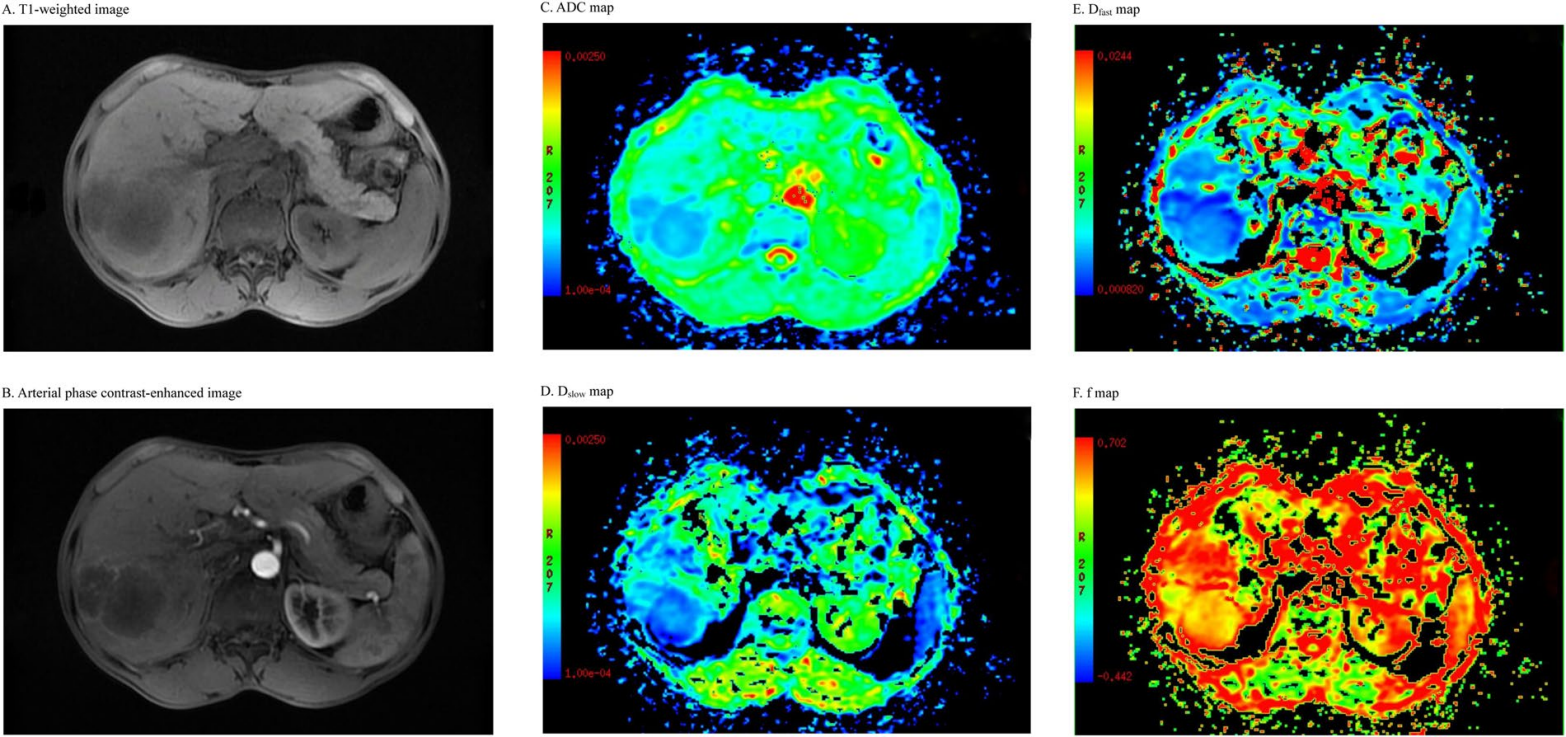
Axial MRI images of an HCC patient. (A): A T1-weighted image shows a hypointensive lesion in the right lobe of the liver. (B): An arterial phase contrast-enhanced image shows an inhomogeneous enhanced lesion. (C): ADC map. (D): D_slow_ map. (E): D_fast_ map. (F): f map.
